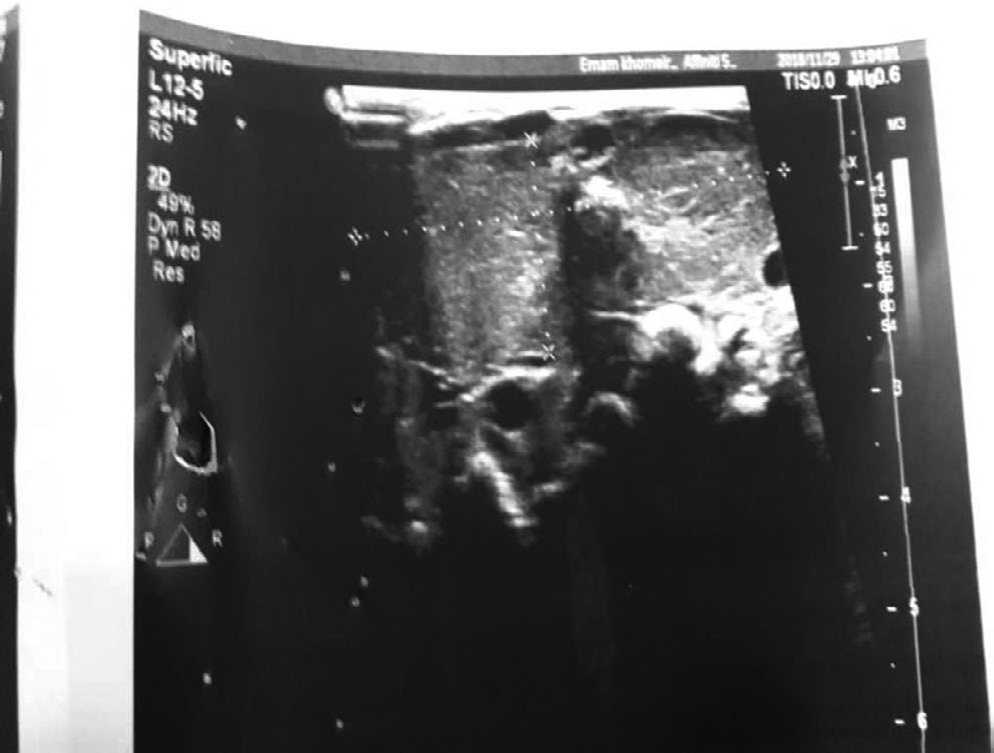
Ultrasound neck mass image
